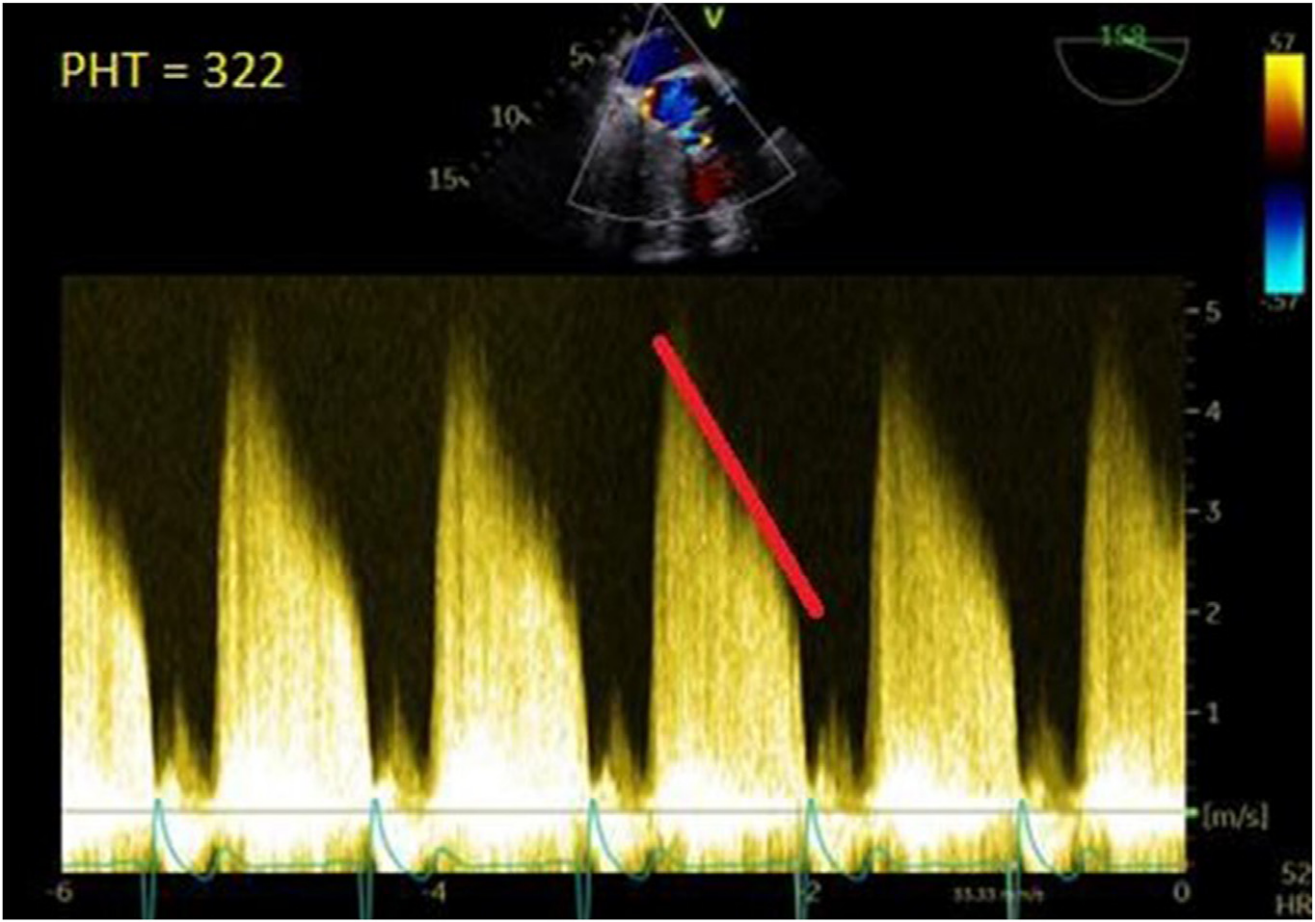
Two-dimensional TEE, mid-esophageal window, long-axis (158°) view with color-flow Doppler-guided continuous-wave Doppler, demonstrates a dense spectral Doppler profile of AR with a short pressure half-time of 322 ms (*red line*).
